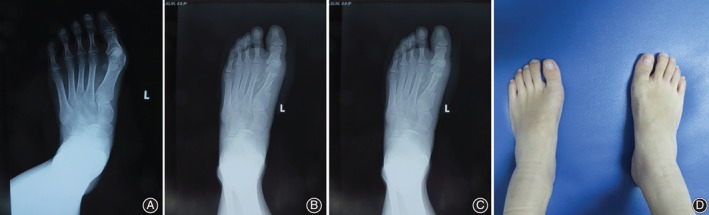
(A) A radiograph of preoperative weight‐bearing position shows hallux valgus deformity. (B) A radiograph of postoperative weight‐bearing position shows adequate correction of hallux valgus deformity. (C) A radiograph of weight‐bearing position 1 year after the surgery. (D) Outward observation of feet 1 year after the surgery.
